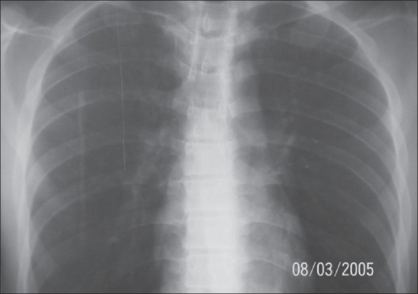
Patient - 2 X-ray chest after granuloma excision
